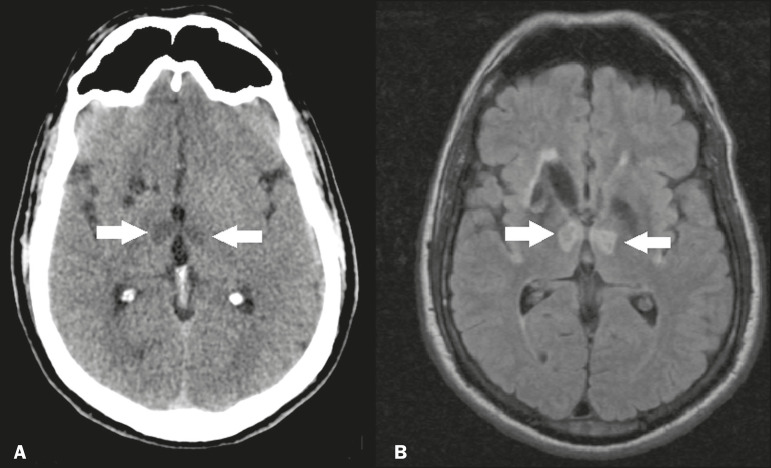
Bilateral thalamic infarcts in a patient with a sudden decrease in the level of consciousness. A: Axial CT slice showing areas of hypodensity in the thalamus (arrows). B: Axial FLAIR MRI sequence showing areas of hyperintensity in the thalamus (arrows).
